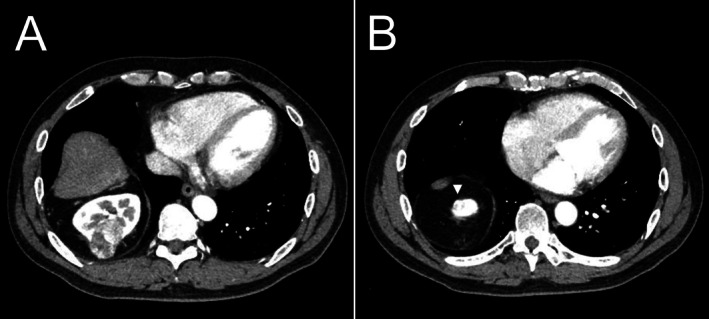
Contrast‐enhanced computed tomography showing two right renal tumors consistent with renal cell carcinoma. (A) A 39‐mm tumor with a R.E.N.A.L. nephrometry score of 8p. (B) A 15‐mm tumor with a R.E.N.A.L. nephrometry score of 4a (indicated by an arrow).
